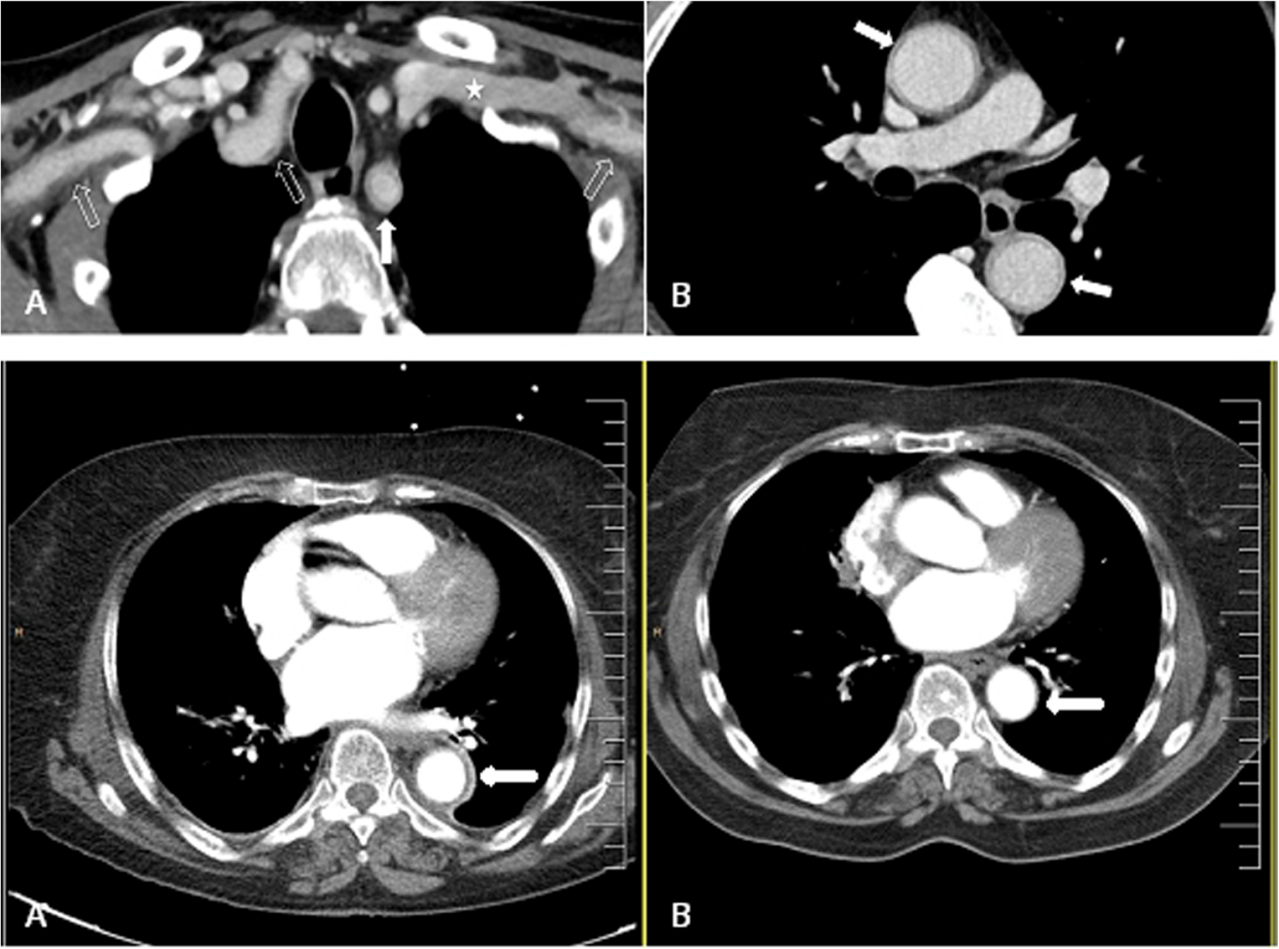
CT angiography of the chest in two patients with GCA. *Upper row* CTA of the aorta and the supra-aortic arteries in a 64-year-old male patient with giant cell arteritis. Mural thickening and contrast enhancement of the aortic wall (arrows in B). Please note hypodense inner ring delineating luminal contrast-enhanced blood from contrast-enhancing thickened aortic wall. Mural inflammatory changes are present in both subclavian arteries as visualized in cross section (bold arrow in A) and in a longitudinal section (light arrows in A). Asterisk in A indicates the left subclavian vein. *Lower row* Axial view of a CT angiography of a 76-year-old woman with GCA showing severely increased wall thickness of 5.2 mm and contrast enhancement of the descending aorta (bold arrow) (A). Contrast CT of the same patient performed 4 years earlier, with no significant aortic wall thickening (B)
